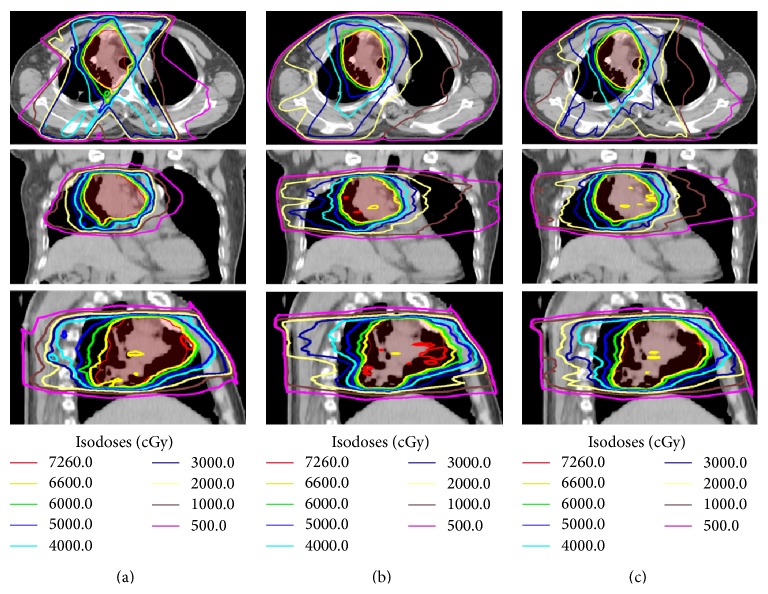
Representative axial, coronal, and sagittal computed tomography slices showing isodose distribution for (a) IMRT, (b) VMAT, and (c) Hybrid IMRT/VMAT. Planning target volume (PTV) shown in red.
